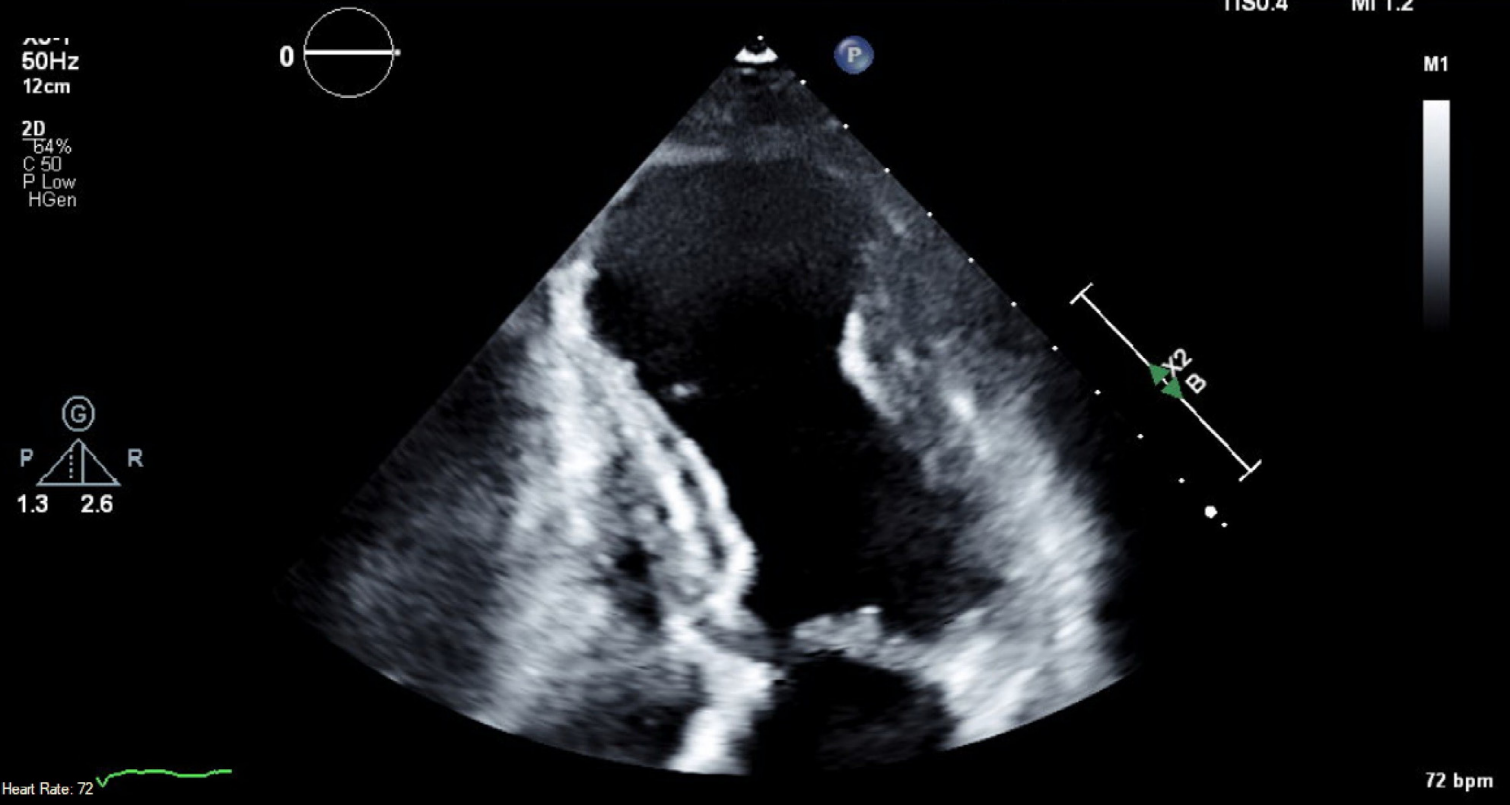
TTE without contrast at the apical 4 chamber view shows resolution of LV thrombi. TTE, Transthoracic echocardiogram; LV, left ventricular
